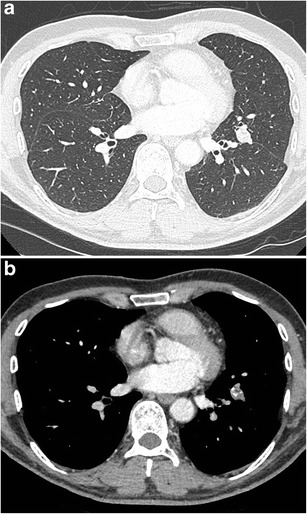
Incidental finding of a solitary pulmonary nodule in a 50-year-old man. Axial CT-image in lung window setting a shows a well-delineated nodule with clear lobulated morphology in the left upper lobe. The absence of growth, internal benign-looking calcifications and clear hypodense areas (corresponding to fat) on the images in mediastinal window setting b led to the probable diagnosis of a hamartoma
